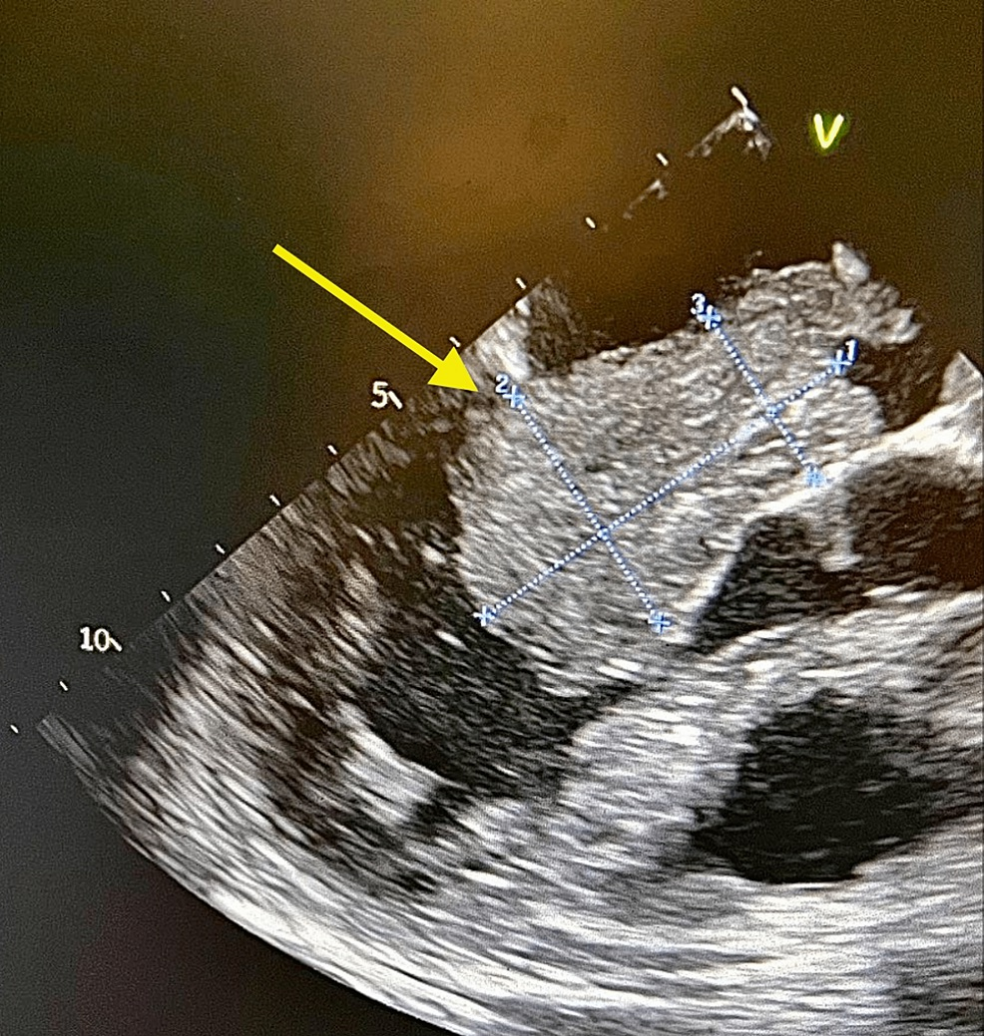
Left atrial myxoma is shown by the yellow arrow. The measured dimensions of the mass in the echocardiogram were 4.9 cm, 3.1 cm, and 2.1 cm indicated by lines 1, 2, and 3, respectively.
